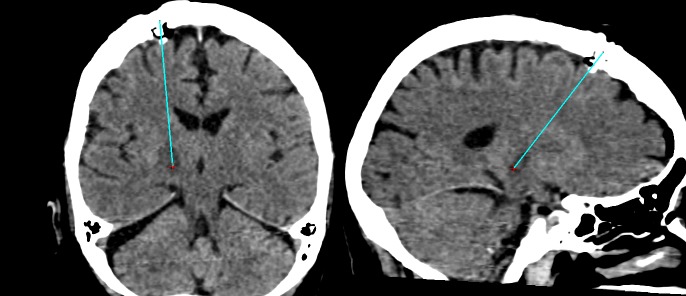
Thalamotomy at the target point and adjacent point The thalamotomy at the right ventro intermedius nucleus is visible as edema surrounding the distal aspect of the probe trajectory depicted by the cyan line in the para-coronal (left) and para-sagittal (right) images. These images are reconstructed from post-operative, non-stereotactic computed tomography (CT) images that are geometrically aligned to pre-operative, stereotactic CT images. The edema is roughly cylindrical because two thermolesions were created 2 mm apart along the probe trajectory. The target point is depicted by the red cross. Compare to Figure 4.
